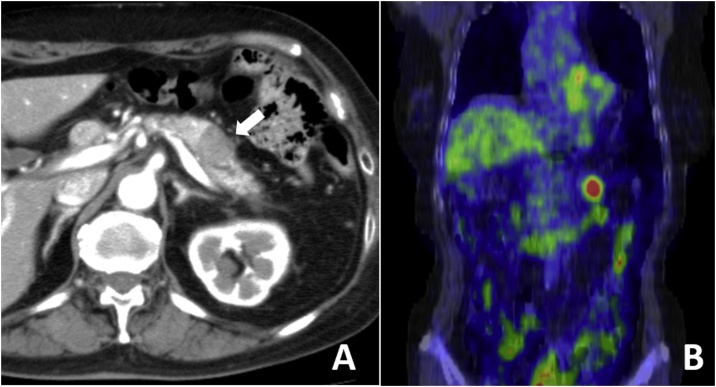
Preoperative findings. (A) Computed tomography reveals a low density mass in the pancreatic tail *(allow).* (B) Positron emission tomography shows a hypermetabolic mass in the pancreatic tail.
